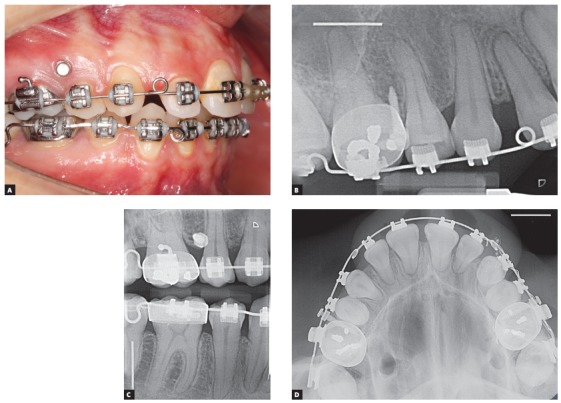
A) Orthodontic mini-implant (OMI) placed between upper second premolar and first molar through the buccal attached gingiva: B) periapical radiograph (PAR); C) vertical bitewing radiograph (VBW); and D) occlusal radiograph (OC).
